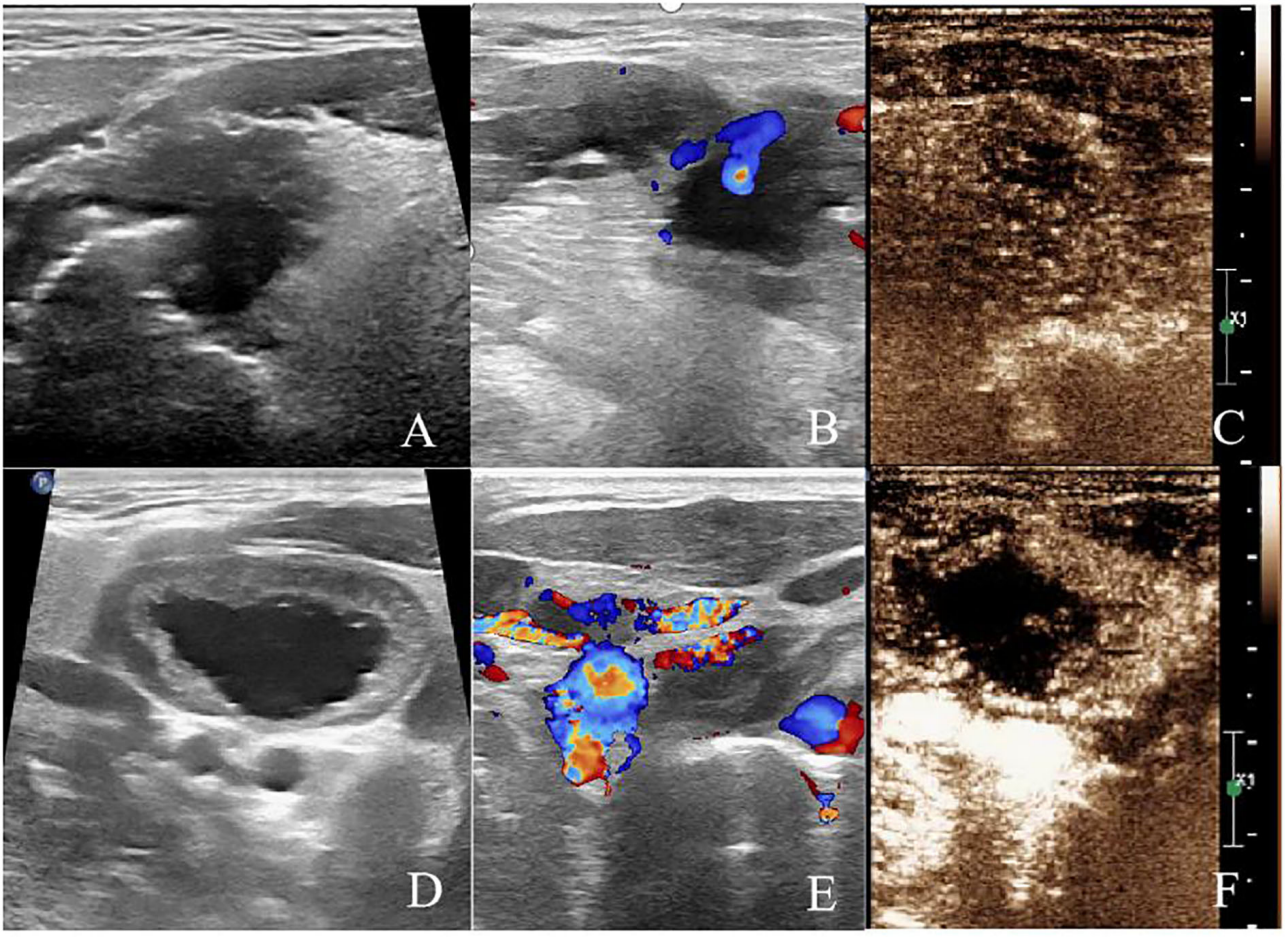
After 2 months of ablation. (A) Two-dimensional ultrasound showed hypoechoic area in the ablation area of the tongue base. (B) CDFI showed no blood flow signal in the ablation area of the tongue base. (C) CEUS showed no enhancement in the ablation area of the tongue base. (D) Two-dimensional ultrasound showed hypoechoic area in the right cervical lymph node (level II). (E) Microvascular imaging showed no blood flow signal in the right cervical lymph node (level II). (F) CEUS showed no enhancement in the ablation area of the right cervical lymph node (level II).
